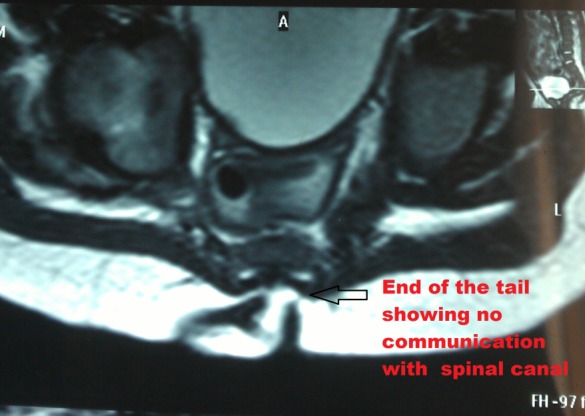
Figure 2:MRI showing no communication with spinal cord.
